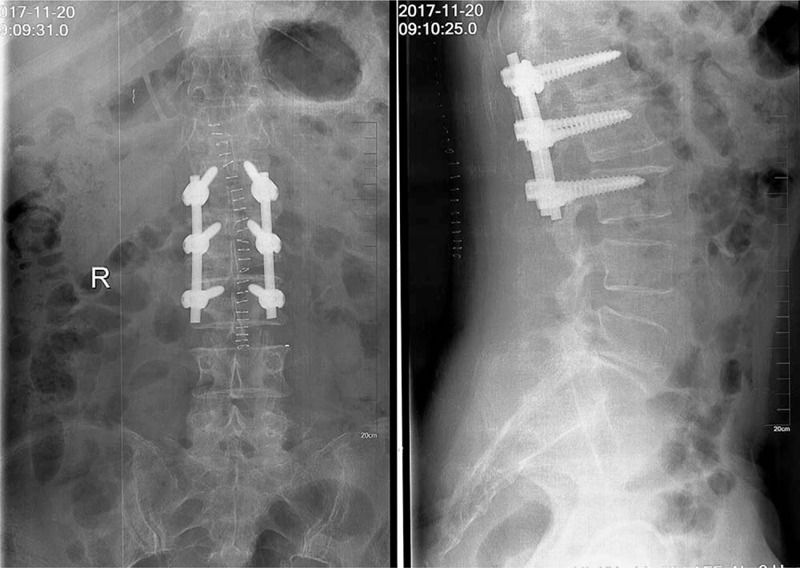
Postoperative radiography and pedicle screw fixation at L1–3.
